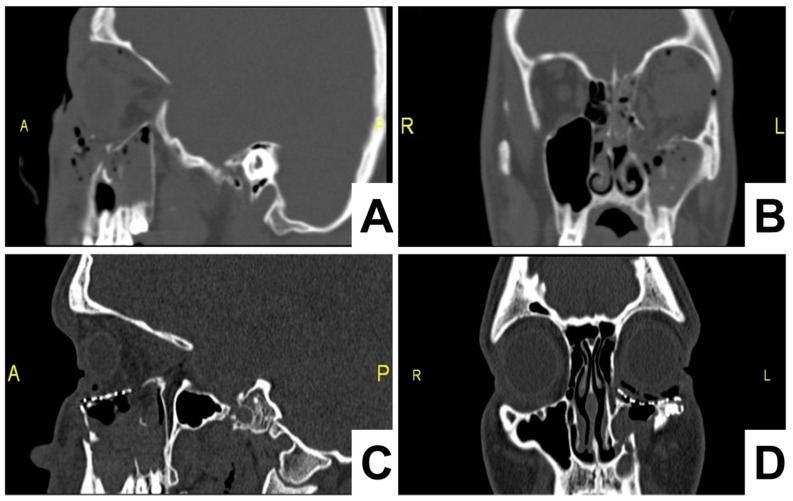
Pre- (A,B) and postoperative (next day) (C,D) control computer tomography (CT) scan of the eyelids in the patient undergoing orbital floor fracture treatment by a fully covered titanium mesh with collagen membrane. A benefit of the titanium mesh is that it does not present artifacts in CT or magneitc resonance (MR, Magnetic resonance) imaging.
